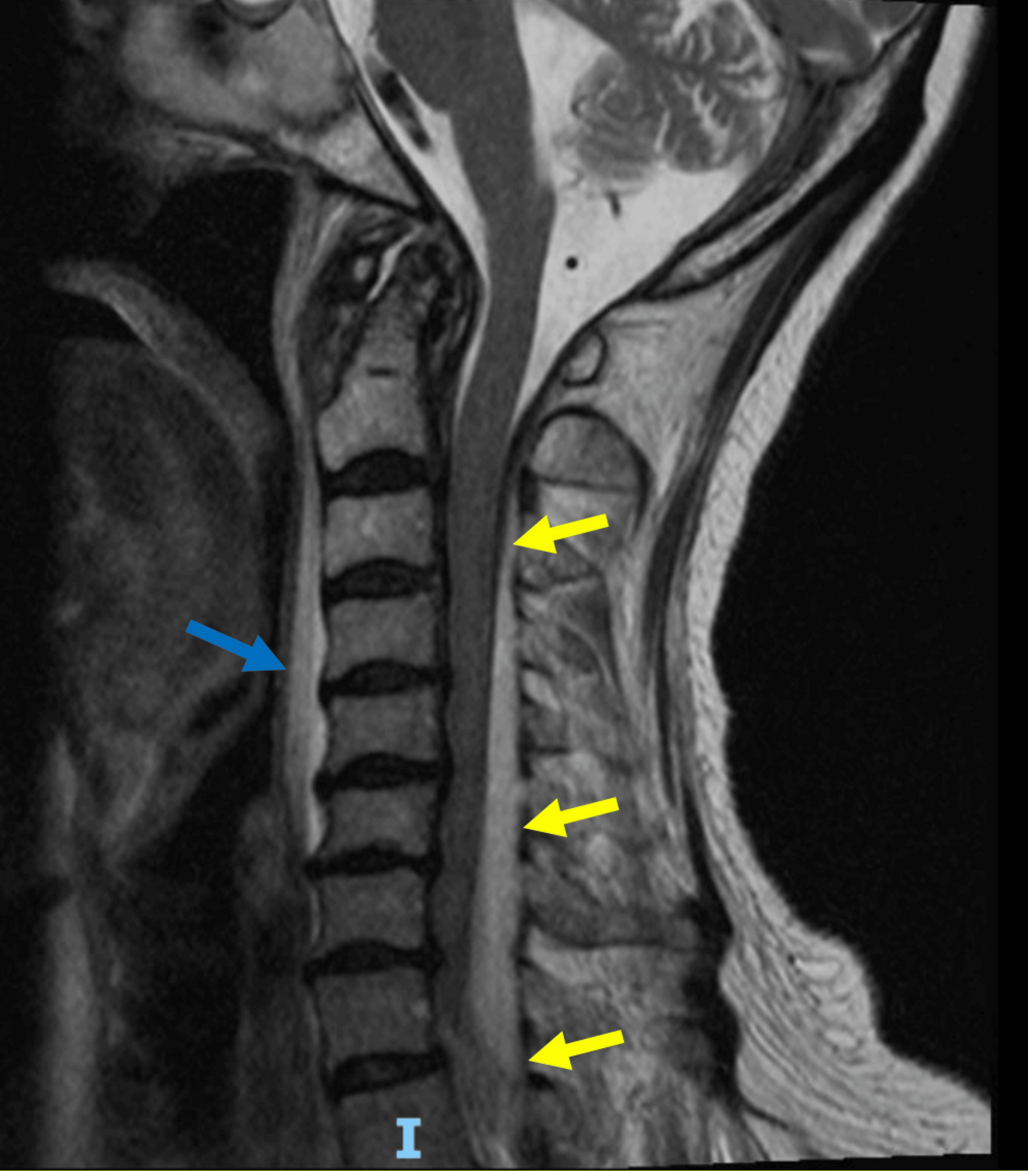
MRI T2-weighted image of the cervical spine (sagittal view) Rim-enhancing collection involving the dorsal epidural space, spanning from C2 level to inferior T1 level with mass effect on the thecal sac and cord most severely within the lower cervical spine (yellow arrow). Rim-enhancing lesion centered within the left longus coli musculature and associated extensive edema of the longus coli muscle and within the retropharyngeal space (blue arrow). MRI: magnetic resonance imaging
